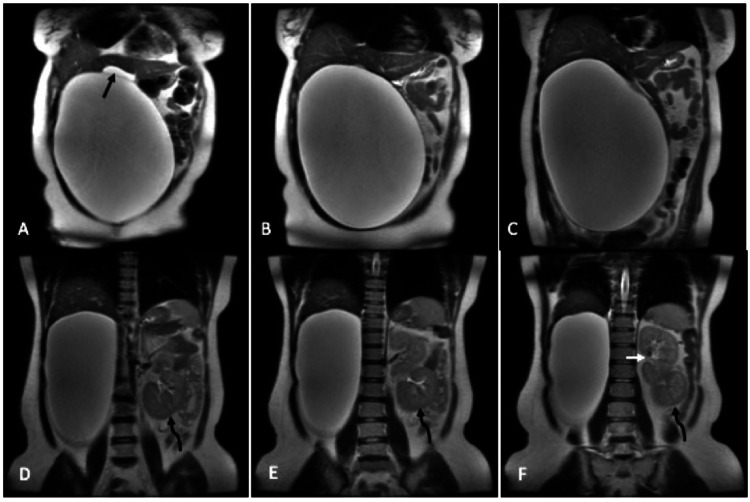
T2W coronal MRI images of the abdomen reveal a giant cyst extending from the right sub-hepatic region upto the iliac fossa and bulging across the midline with compression and displacement of the surrounding structures The black arrow in A shows the superiorly displaced and compressed gall bladder and curved black arrows in D, E, and F point toward the displaced right kidney into the left lumbar region below the left kidney (white arrow in F).
